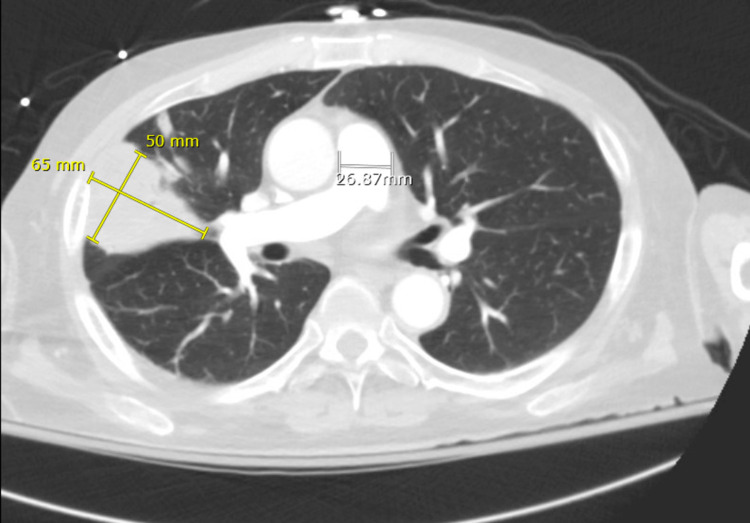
Axial view of CT chest also demonstrating a dominant mass in the right upper lobe, measuring up to 6.5 x 5.0 cm.
